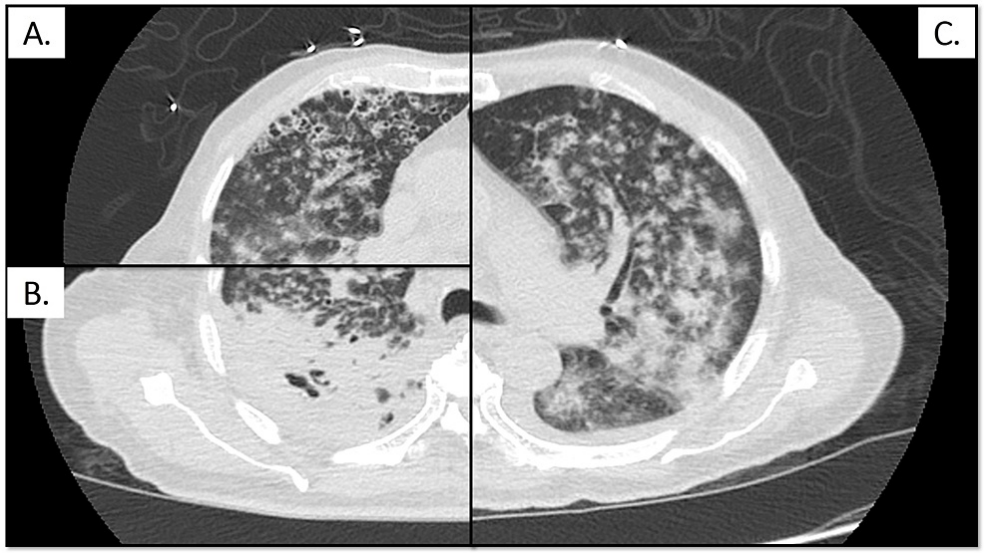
Representative sections from axial CT of the thorax showing A) thin-walled cystic changes, B) cavitary consolidation, and C) diffuse miliary nodules with surrounding ground glass in different areas of the lung, all of which can be seen in disseminated coccidioidomycosis.
